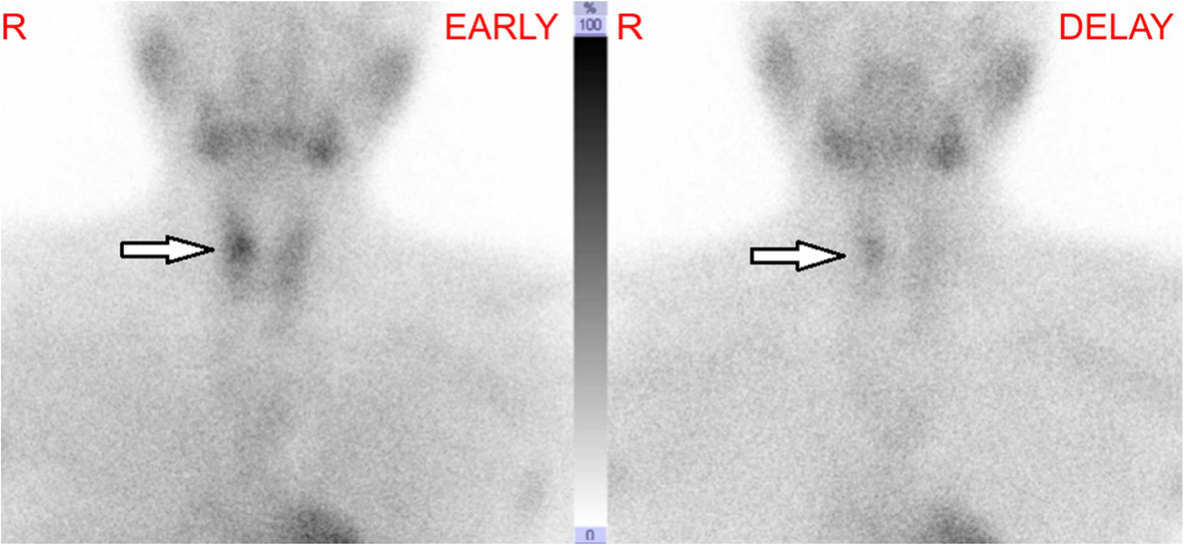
Technetium-99m methoxy-isobutyl isonitrile (Tc99m MIBI) scintigraphy. This image shows accumulation at the same site that had been identified through ultrasonography.
